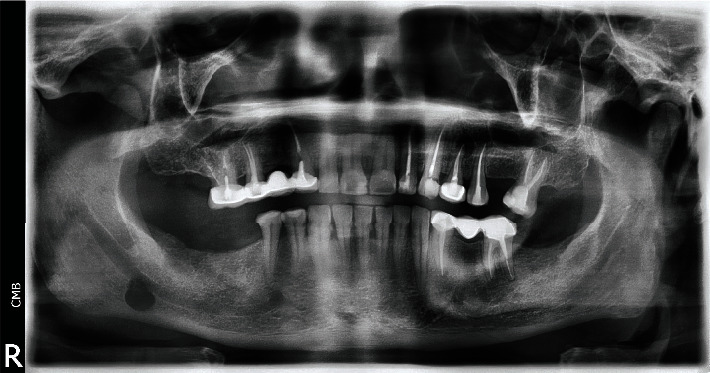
Panoramic radiograph showed a well-limited radiolucency image with a clear peripheral regular condensation border, located below the mandibular canal.
